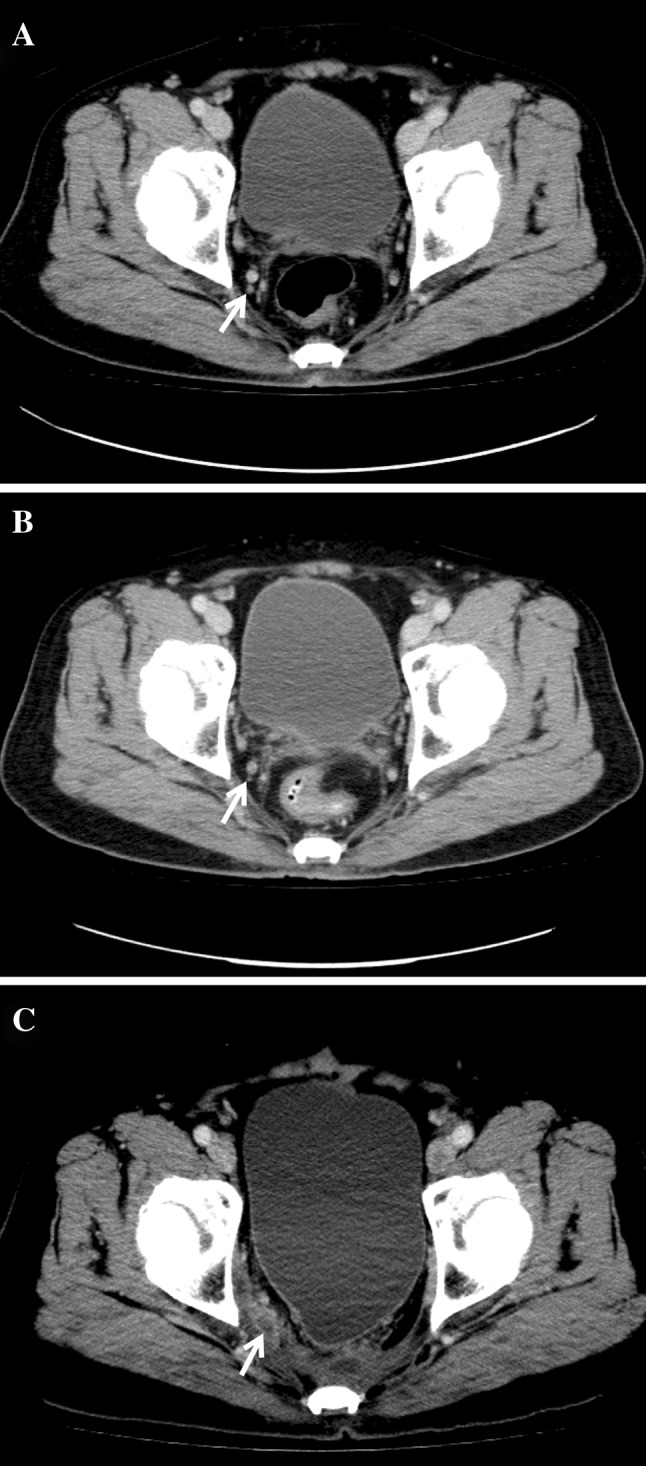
Lateral pelvic recurrence on computed tomography. a Pre-treatment image shows a 5-mm lymph node in the right obturator area (*white arrow*). b Patient underwent neoadjuvant chemoradiotherapy and the metastatic lymph node was decreased to 2 mm (*white arrow*). c Follow-up image at 12 months after surgery shows lateral pelvic recurrence (*white arrow*).
